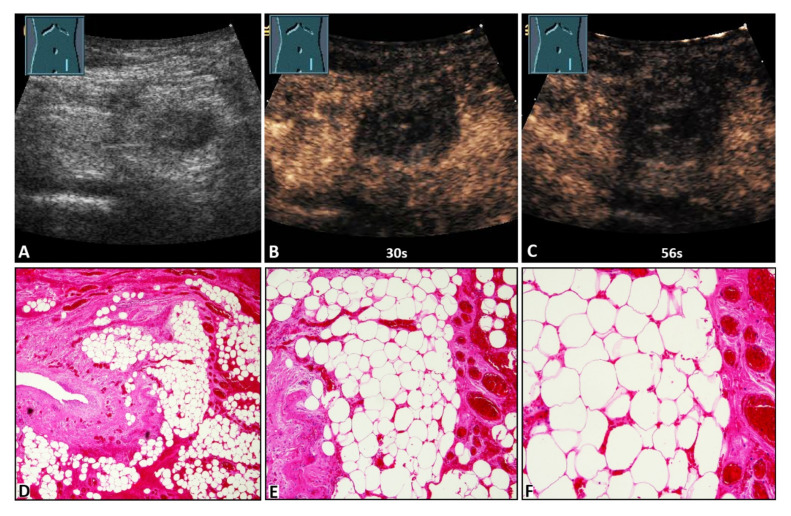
A 47-year-old female patient with acute peritoneal pain in the left lower abdomen and a palpable inguinal-located tumor. (A) B-mode ultrasound shows a hyperechoic lesion with a central hypoechoic area. (B,C) On contrast-enhanced ultrasound, the lesion shows absent enhancement after 30 s and 56 s, and an omental hernia was diagnosed. (D) Omentum with partial fibrotic consolidation (on the left) and dilated hyperemic capillaries and hemorrhages (4× magnification). (E) Omentum with central necrotic fat tissue (missing nuclei), fibrosis (to the left), and dilated hyperemic capillaries and hemorrhages (on the right) (10× magnification). (F) Omentum with central necrotic fat tissue, and dilated hyperemic capillaries and hemorrhages (20× magnification).
